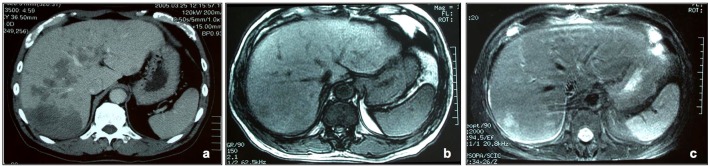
Radiological findings of the liver. (a) Enhanced CT of delayed phase. Multiple low density areas are detected in the whole liver. The lesions show nodular or geographic, and no enhanced effects. (b, c) MRI images. These lesions are iso or low intensity in T1-weight image (b), and iso or hyper intensity in T2-weight image (c).
